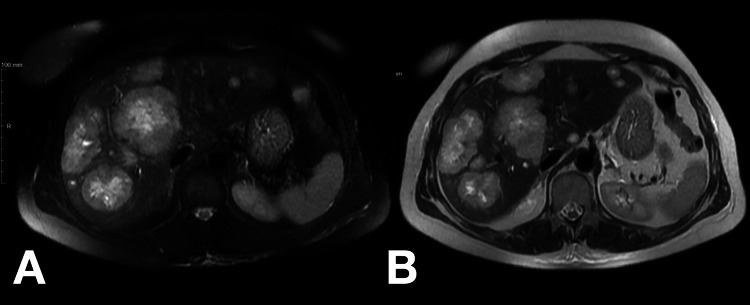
Abdominal MRI showing multiple nodules compatible with liver metastasis T2 fat-suppressed (A) and T2-weighted MRI (B) images showing multiple heterogeneous nodules on the liver parenchyma consistent with metastatic lesions MRI: magnetic resonance imaging
